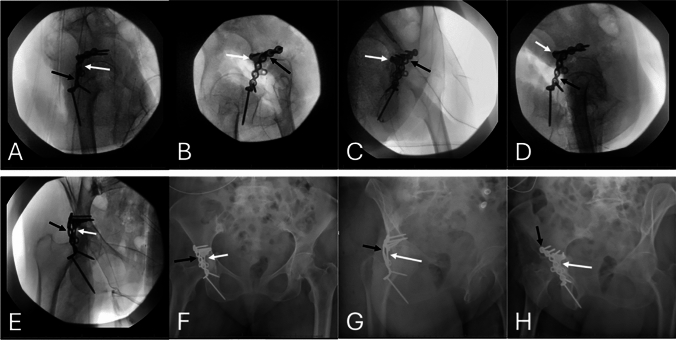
Intraoperative fluoroscopic images A-E demonstrating reduction and fixation of a posterior wall acetabular fracture. The H-plate (white arrows) was positioned to buttress the posterior wall fragment for primary stabilization. A 7-hole reconstruction plate (black arrows) was contoured and applied over the H-plate (white arrows) to provide supplemental fixation for an associated column fracture and buttress support of the posterior wall. F–H Postoperative radiographs of the pelvis, including anteroposterior (AP) view F, obturator oblique view G, and iliac oblique view H. Images demonstrate appropriate hardware positioning, restoration of posterior column alignment, and joint congruity following fixation of the posterior wall acetabular fracture
